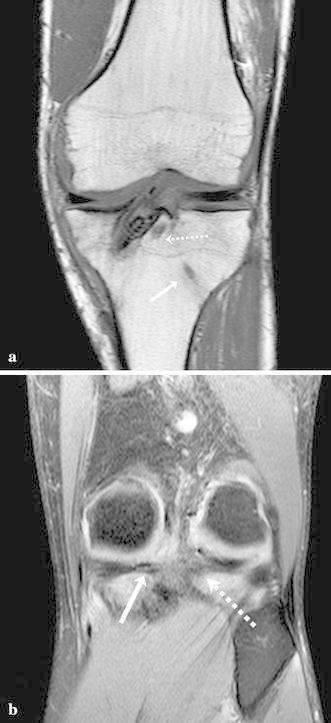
At MRI follow-up at three months, the coronal T1-TSE view (a) shows the tibial tunnel for ACL reconstruction and the tunnels for the medial root (*white arrow*) and for the lateral root (*dashed arrow*). b The coronal TSE-fat saturated MR image shows both medial and lateral roots healed
